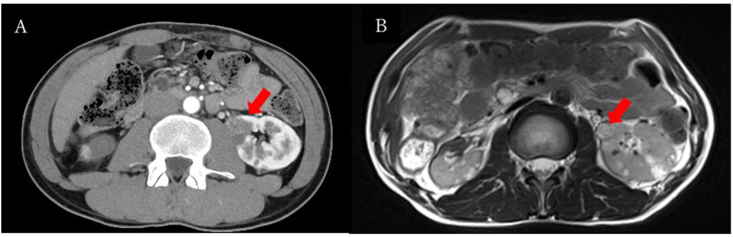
Dynamic computed tomography (A) and T2-weighted magnetic resonance imaging (B) revealing remaining multiple renal tumors in the left kidney (largest diameter, 10 mm [arrow)] at 3 years after surgery for right renal tumors.
